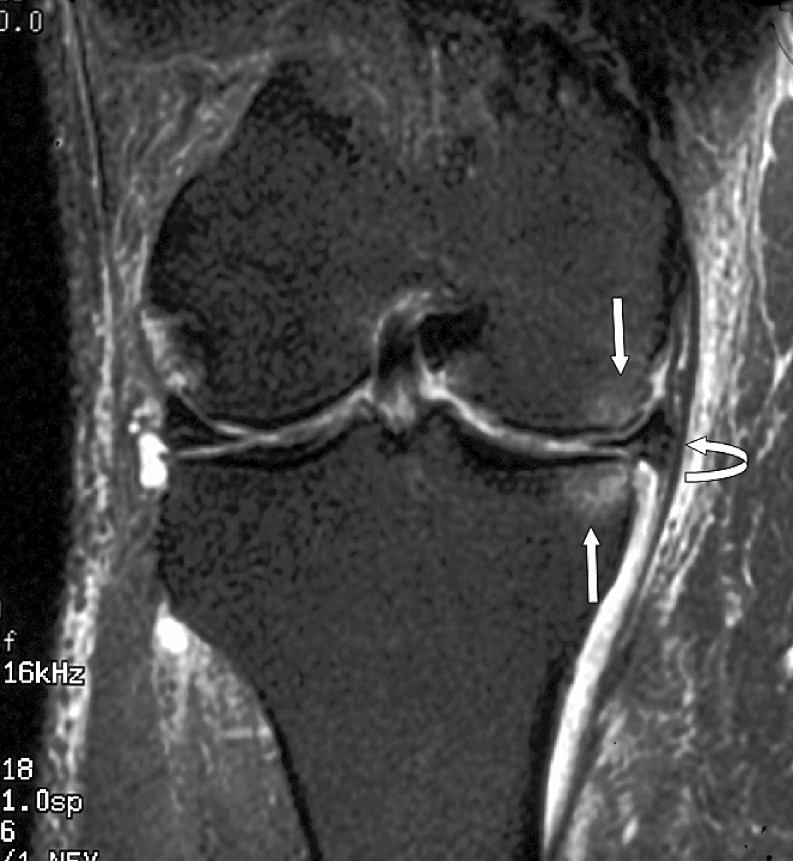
Focal edema, no total knee joint replacement, in a 58-year-old male patient. Coronal fast fat suppressed T2-weighted image (TR 3000/TE 56/256 × 192) shows extrusion of the medial meniscus (*curved arrow*) and small adjacent areas of focal marrow edema (*straight arrows*). The medial meniscus has a degenerative horizontal cleavage tear approximating the tibial surface. Note also the moderate loss of hyaline cartilage
